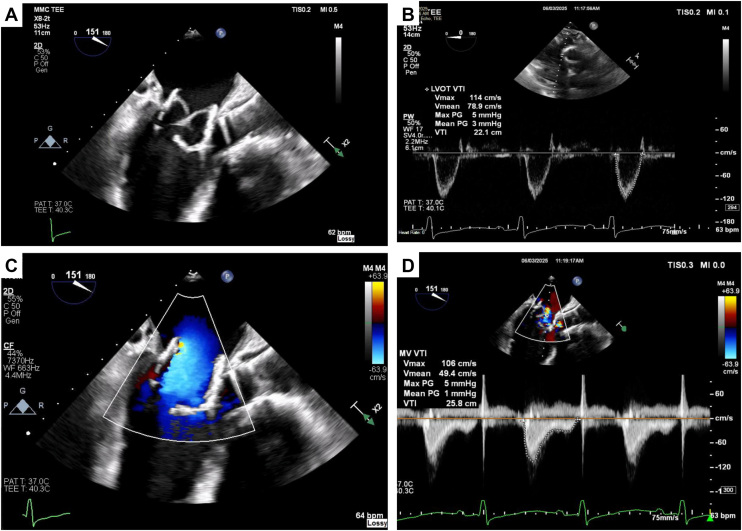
Final transvalvular gradients. (A) Valve-in-Ring TMVR on 2D TEE. (B) LVOT mean gradient of 3 mm Hg. (C) Valve-in-Ring TMVR on color TEE. (D) Mean transmitral valve gradient of 1 mm Hg. AML, anterior mitral leaflet; LVOT, left ventricular outflow tract; TEE, transesophageal echocardiography; TMVR, transcatheter mitral valve replacement.
